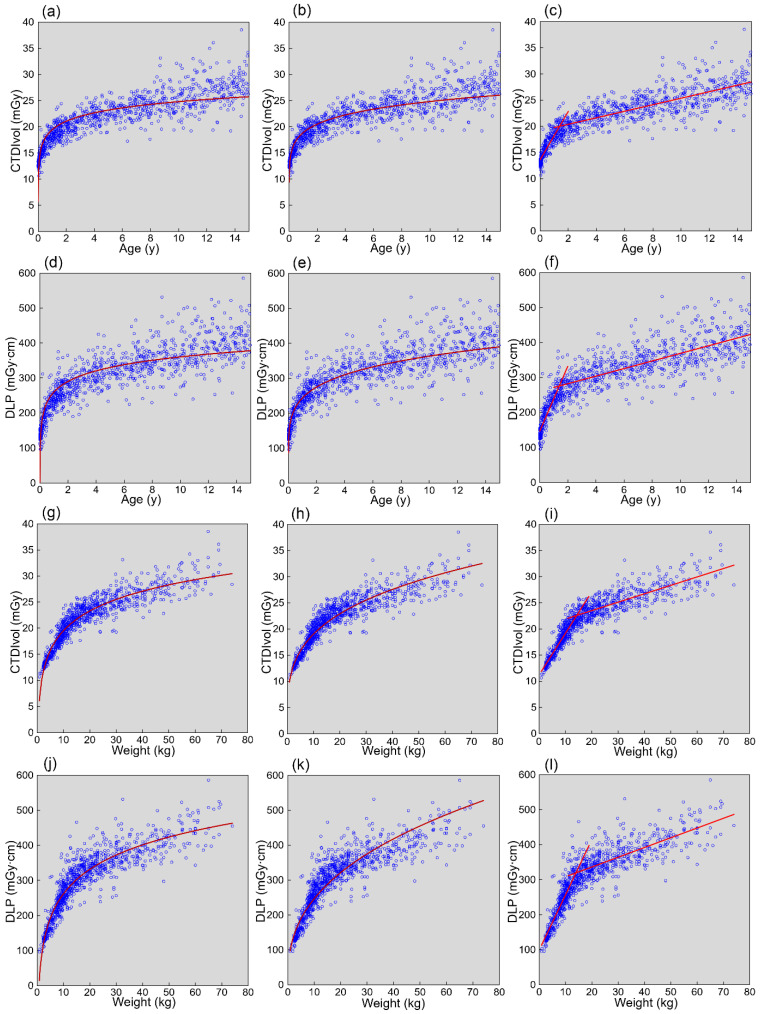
Relationships between CT dose indices and age or weight. Plots of CTDIvol versus age (a–c), DLP versus age (d–f), CTDIvol versus weight (g–i), and DLP versus weight (j–l) are presented. The red lines represent the fitting functions. Logarithmic (a,d,g,j), power (b,e,h,k), and bilinear functions (c,f,i,l) were used for fitting.
